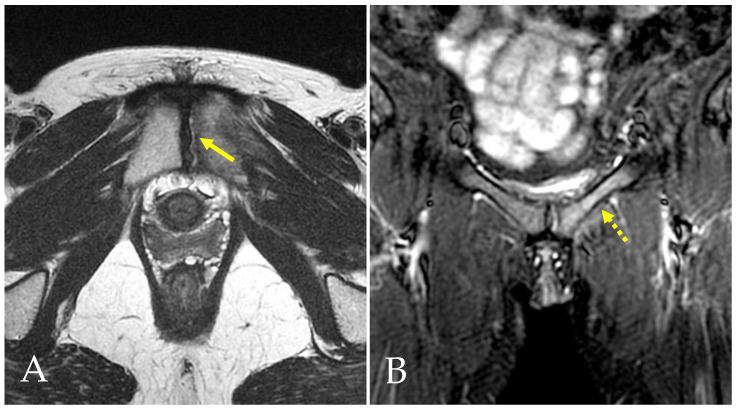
Axial T2 (A) and coronal STIR (B) MR images showing stress fracture as a linear subchondral hyperintensity (arrow). Coronal STIR image also shows diffuse BME of left pubic ramus (dotted arrow).
